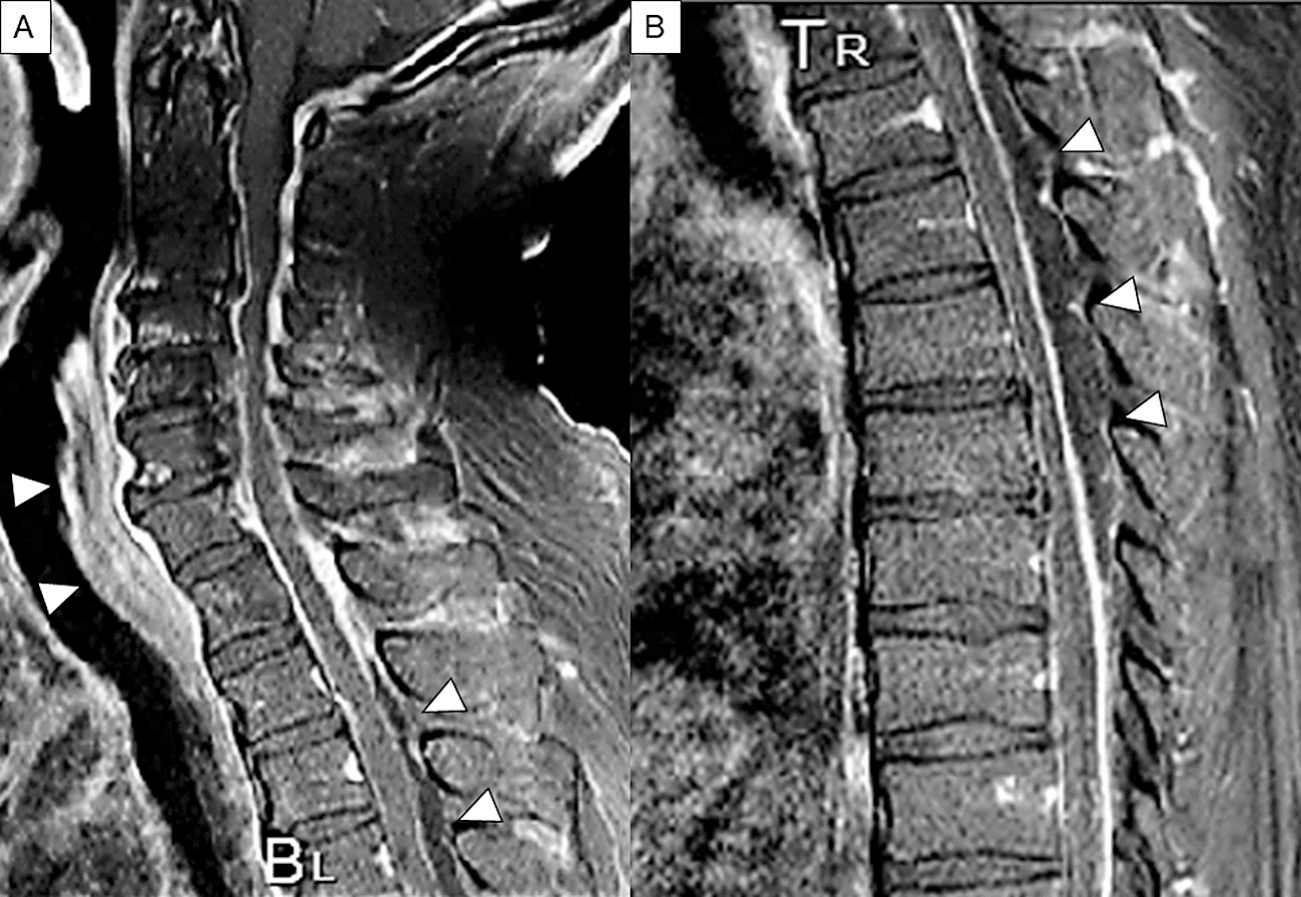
Sagittal Gd-enhanced T1-weighted MRI. Spinal epidural abscess is noted that a capsule-like rim whose edges are strongly enhanced and the content of abscess shows low intensity in the cervical (A) and thoracic (B) spine. The retro-pharyngeal abscess is also noted as enhanced mass (A). Arrowheads indicate the abscess.
